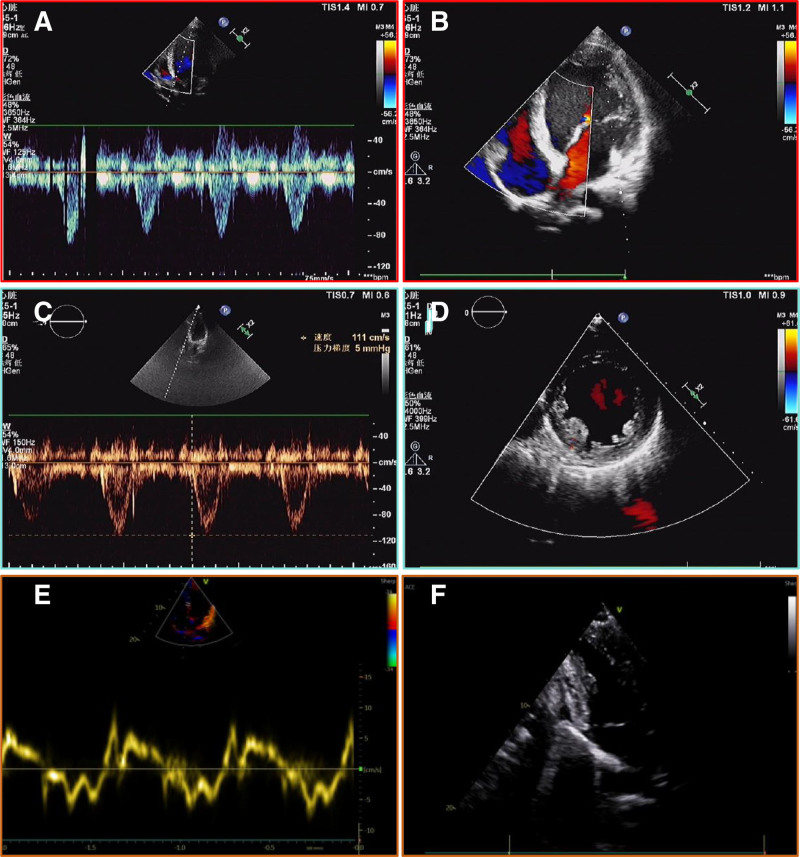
Echocardiogram images at different times. (A, B) on Nov.9, 2020, decreased centripetal motion of the walls of the left ventricle and uncoordinated ventricular wall motion, enlarged left heart, regurgitation at all valves, reduced systolic function; pericardial effusion. (C, D) On Feb.22, 2021: left ventricular myocardial changes; enlarged left heart; trace regurgitation of the bicuspid and tricuspid valves; reduced left ventricular systolic and diastolic function; trace pericardial cavity effusion. (E, F) on Dec 4, 2023: no abnormalities in left ventricular systolic and diastolic function.
